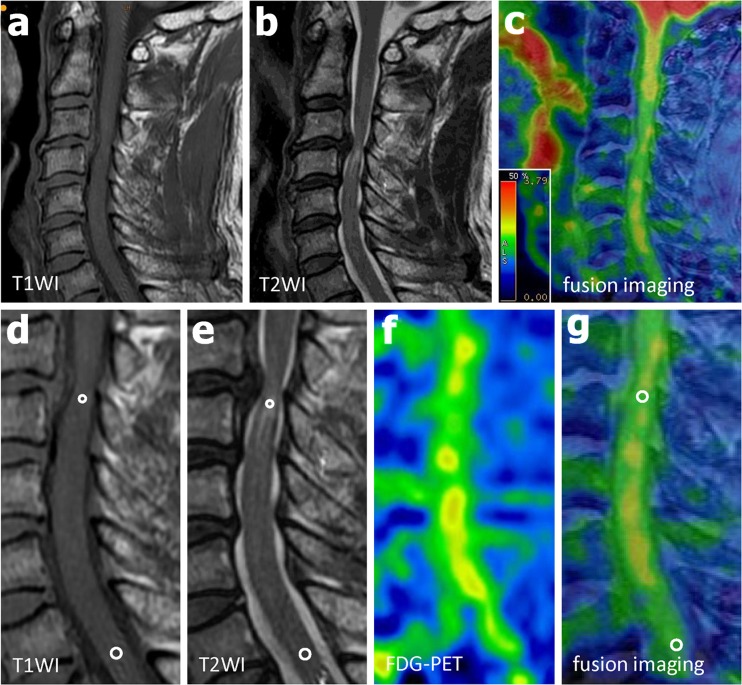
Patient 16. A 62-year-old man with cervical spondylotic myelopathy was treated surgically and showed a poor neurological improvement rate of 28.6 % at follow-up. a Midsagittal T1-W MR image. b Midsagittal T2-W MR image. c MRI/PET fusion image. d T1-W MR image for calculation of SIR as the ratio of the signal intensity in the midsagittal area of increased signal intensity (*small circle*) to the signal intensity in the midsagittal area of normal signal intensity at the level of the C7/T1 disc (*larger circle*), e T2-W image for calculation of SIR in a similar manner. f Sagittal ^18^F-FDG PET image. g Sagittal MRI/PET fusion image demonstrates inconspicuous ^18^F-FDG uptake at the level of a lesion showing increased signal intensity. SUVR is calculated as the ratio between the SUV_max_ of the lesion (*circle* diameter 4 mm) and the SUVmean of the normal cord at the level of the C7/T1 disc (*circle* diameter 4 mm)
